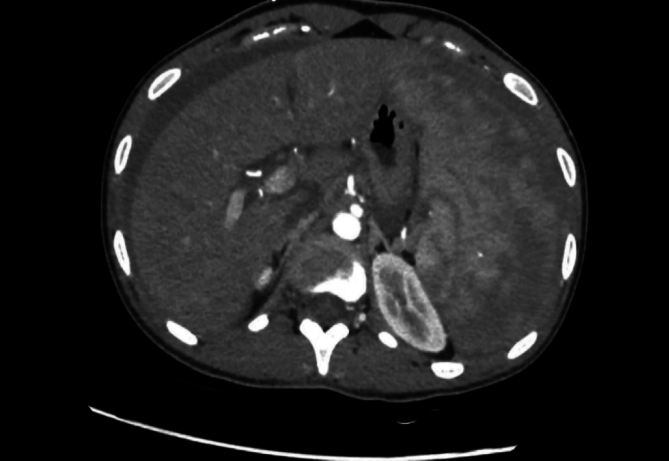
Abdominal CT-scan (axial view).
